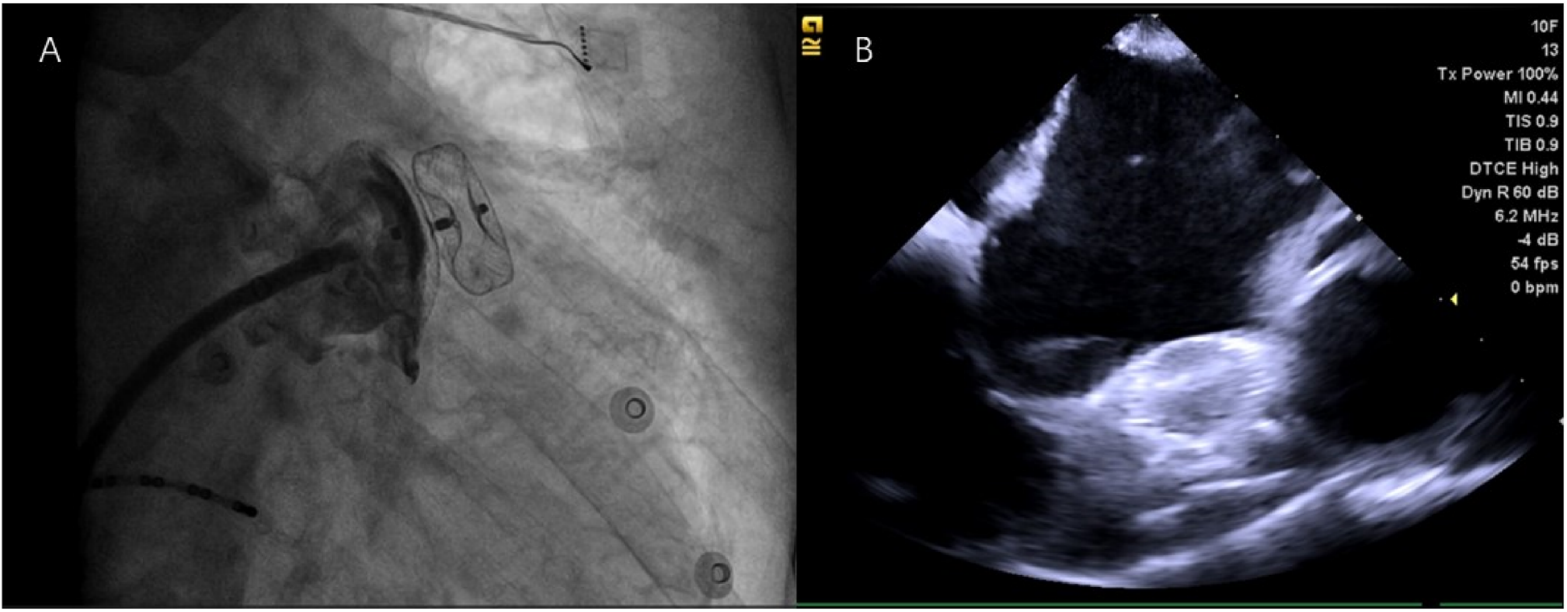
No peridevice leaks were detected after the procedure, as shown in x-ray (A) and intracardiac echocardiography (B).
